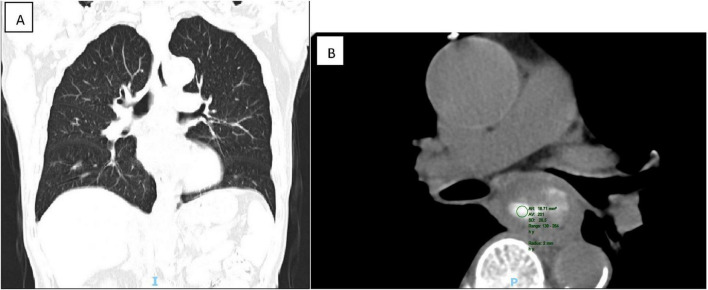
CT showed that the wall of the middle esophagus was uneven and significantly thickened, corresponding to the stenosis of the lumen. The middle esophagus cancer was considered (A: coronal view, B: transverse view).
